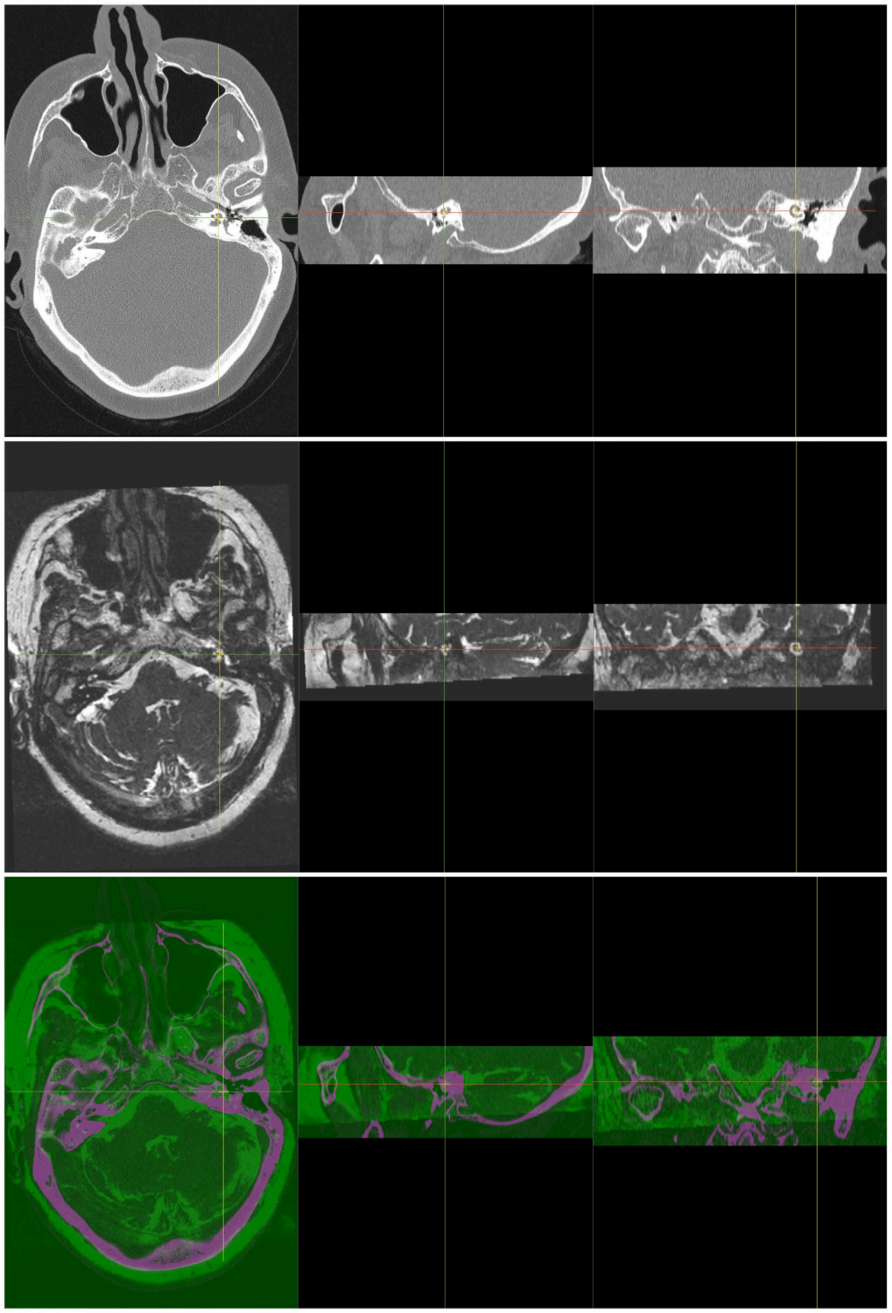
Two input 3D cochlea images of the same patient, CT as a fixed image (top) and MR as a moving image (middle). The bottom image is the registered and fused 3D image, with magenta colour representing the MR part and green colour representing the CT part. Each image has 3 views, from left to right: axial, sagittal and coronal.
